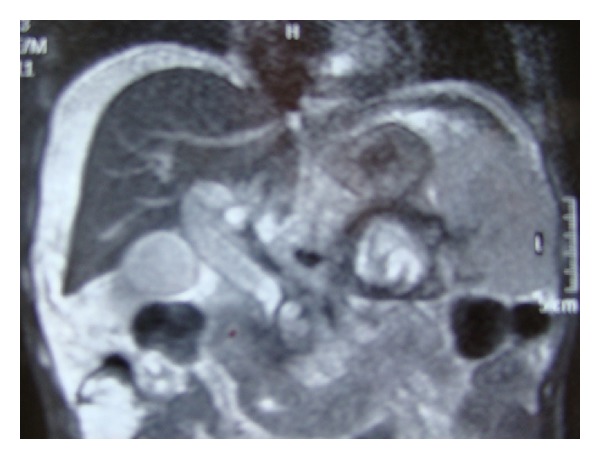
Preoperative magnetic resonance image showing retropancreatic collection, partial necrosis of the pancreatic body, normal liver appearance, and ascites.
